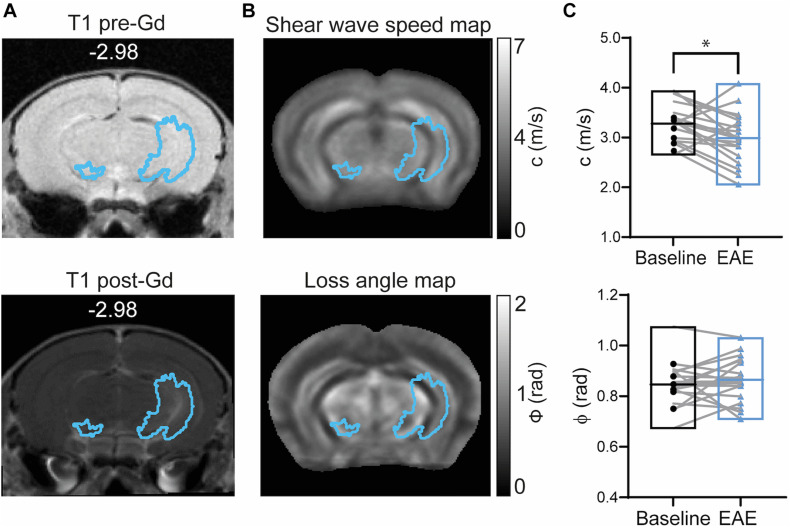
Brain mechanical properties in Gd-enhanced lesions during EAE. (A) Representative T1-weighted image acquired before (left) and after GBCA (right) injection (corresponding GBCA mask overlaid) shows Gd-enhancing lesions near the left hippocampal artery. (B) GBCA mask overlaid on averaged MRE maps of stiffness (shear wave speed) and fluidity (loss angle). (C) Stiffness represented by shear wave speed (c) was reduced during EAE (*p* = 0.0183; paired *t*-test) whereas tissue fluidity (ϕ) was unchanged (*p* = 0.3683; paired *t* test). *n* = 19; mean, min/max; *<0.05. Of note, ventricles are excluded from our analysis since MRE cannot be performed in fluid compartments.
